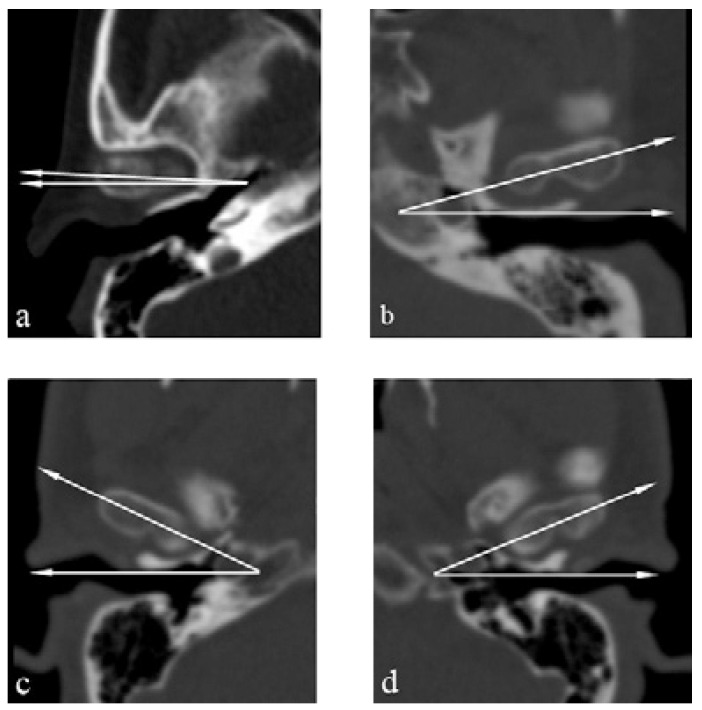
Axial CT scans of the patients revealed the following angles between the axis of the condyle and the transverse plane. a) 3º (Patient-5, right side), b) 15º (Patient-1, left side), c) 25º (Patient-6, right side), d) 22º (Patient-6, left side).
